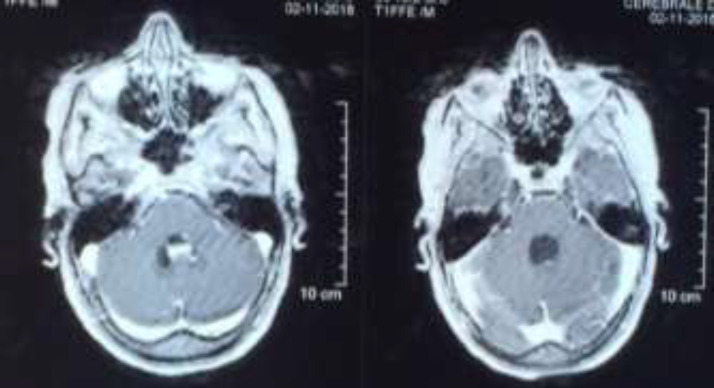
Axial section of a brain MRI on T1-weighted image with contrast injection revealing a cystic lesion of the 4th ventricle, associated to a nodular enhancement of the central and lateral apertures and of the fifth cranial pair
